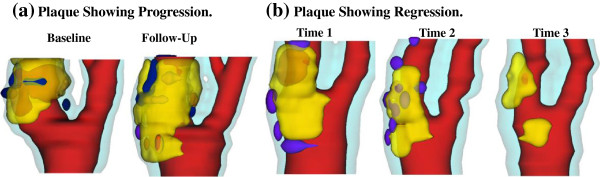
3D plaque samples re-constructed from *in vivo *MR images showing progression and regression. (a): one sample showing plaque growth; (b): one sample showing plaque reduction. Scan time interval: 18 months. Red: lumen; Yellow: lipid; Dark blue: calcification; light blue: outer wall.
